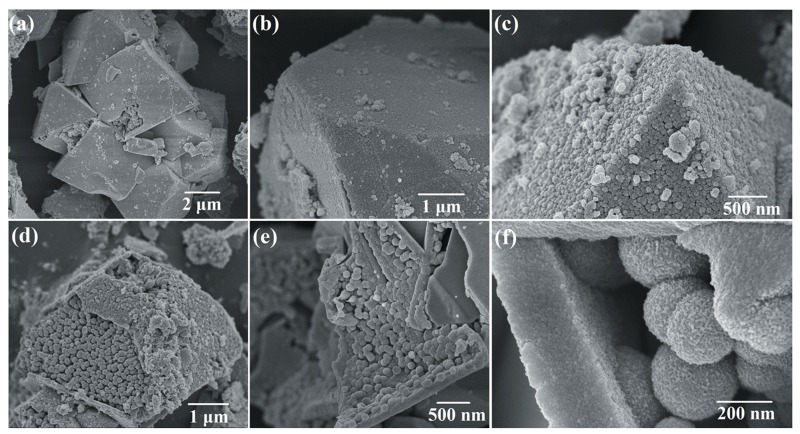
The SEM images of (a,b) S1 and (c) S2, the SEM images of (d,e) S1, (f) S2 treated by ultrasonic.
